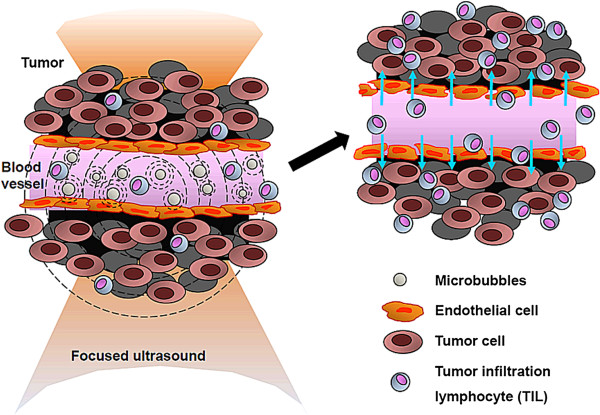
Model of immunological response triggered by microbubble-facilitated focused ultrasound exposure. Exposure of tumor tissue to FUS in the presence of MBs significantly enhances blood-vessel permeability, changing the microenvironment of the tumor tissue region, and enhancing the recruitment and penetration of tumor-infiltrating lymphocytes into the tumor tissue.
